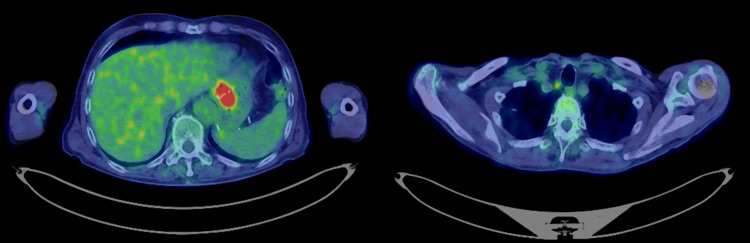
Positron emission tomography/computed tomography Positron emission tomography/computed tomography revealed a primary lesion at the esophagogastric junction and an enlarged right cervical lymph node (no. 101R), both showing significant abnormal fluorodeoxyglucose uptake. No distant metastases were observed.
